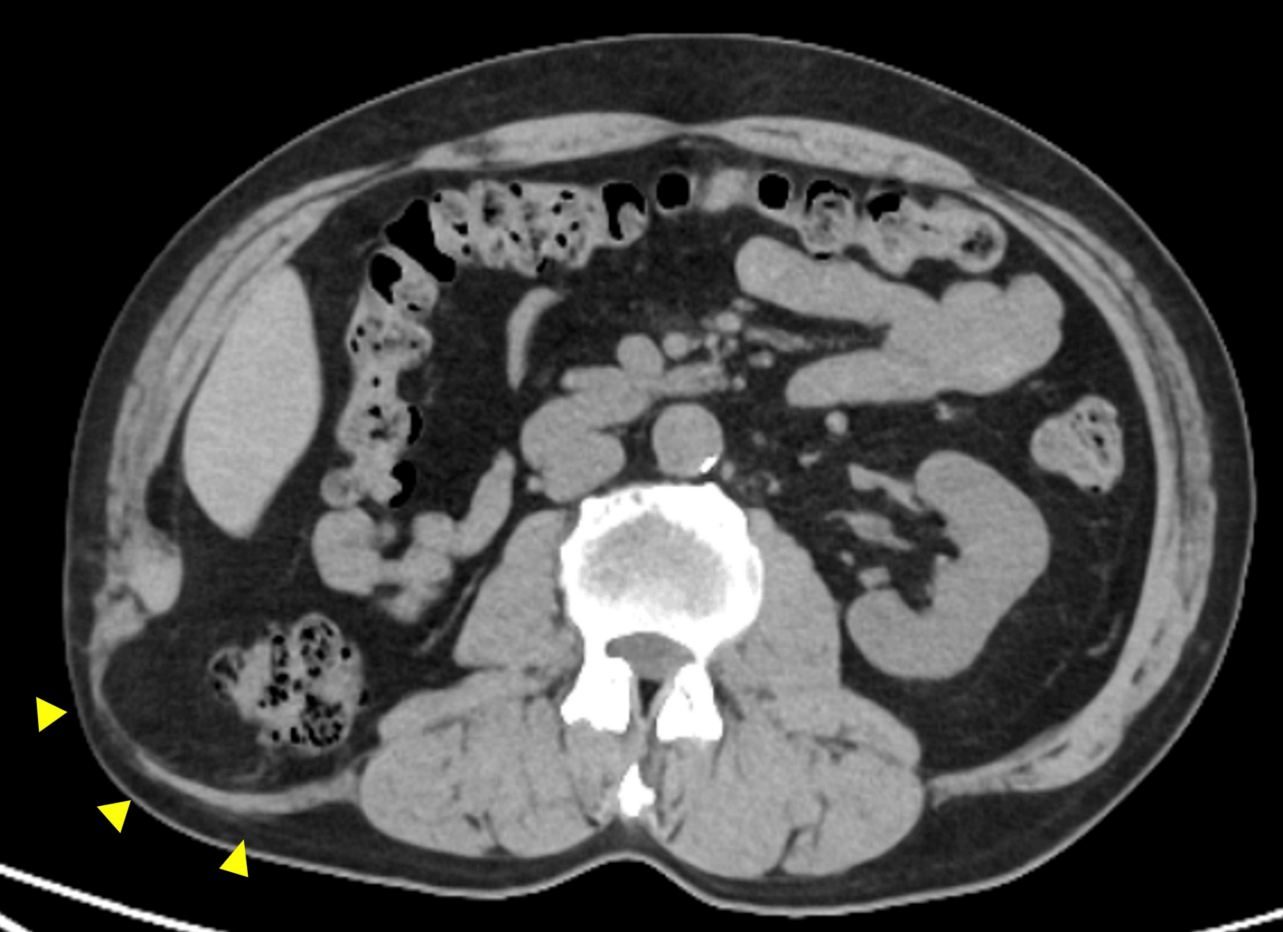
Preoperative computed tomography image of the lumbar hernia The image reveals protrusion of the hernia sac containing retroperitoneal fat tissue and the ascending colon (yellow arrowheads).
